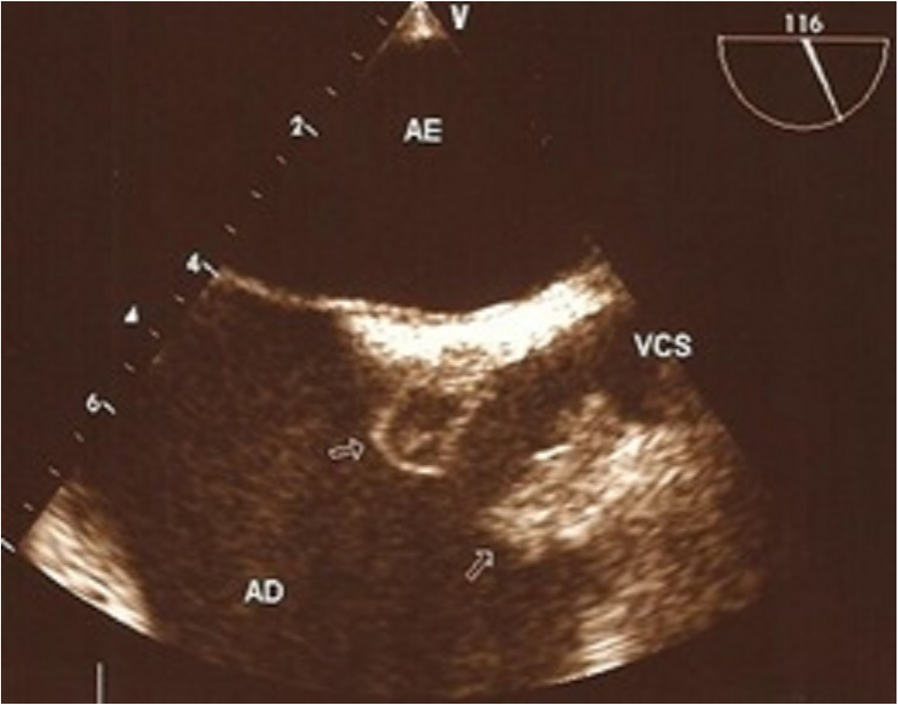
Echocardiography showing two images of sub-endothelial abscesses in the transition from the superior vena cava and right atrium.
